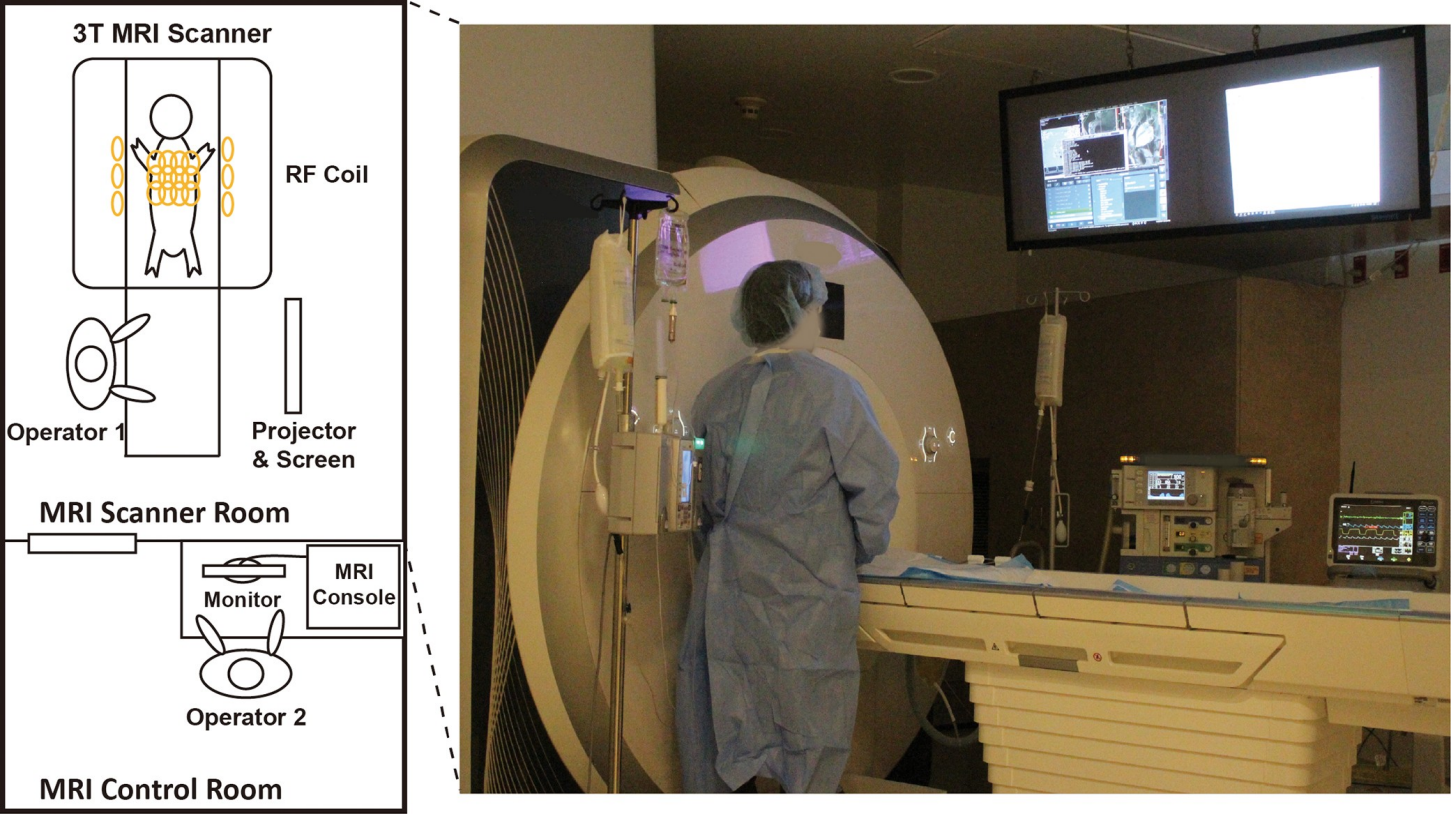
MRI scanner room setup for cardiac catheterization experiments. The guidewire maneuvering was performed by operator 1 in the MRI scanner room and the real-time scan was controlled by operator 2 in the MRI control room.
